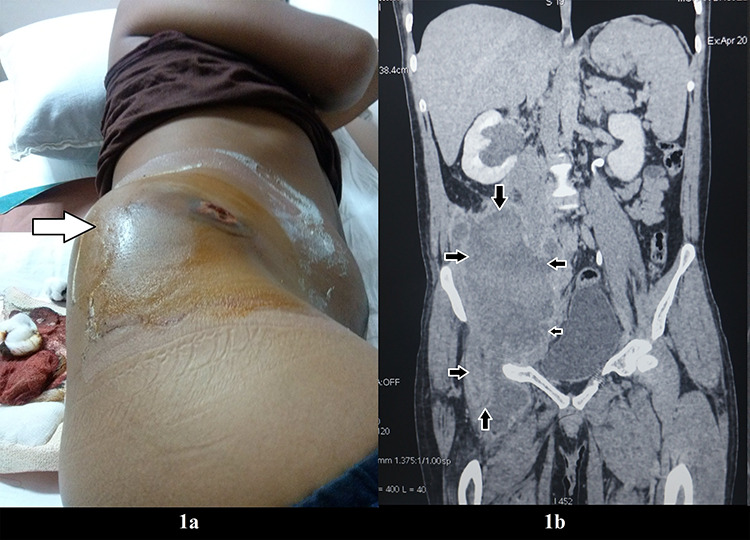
(a) Firm mass in the right groin (indicated by arrow); (b) CT scan of the abdomen showing a large mixed density pelvic mass eroding the right iliac blade with extension to the outer surface of the iliac blade (delineated by small arrows).
